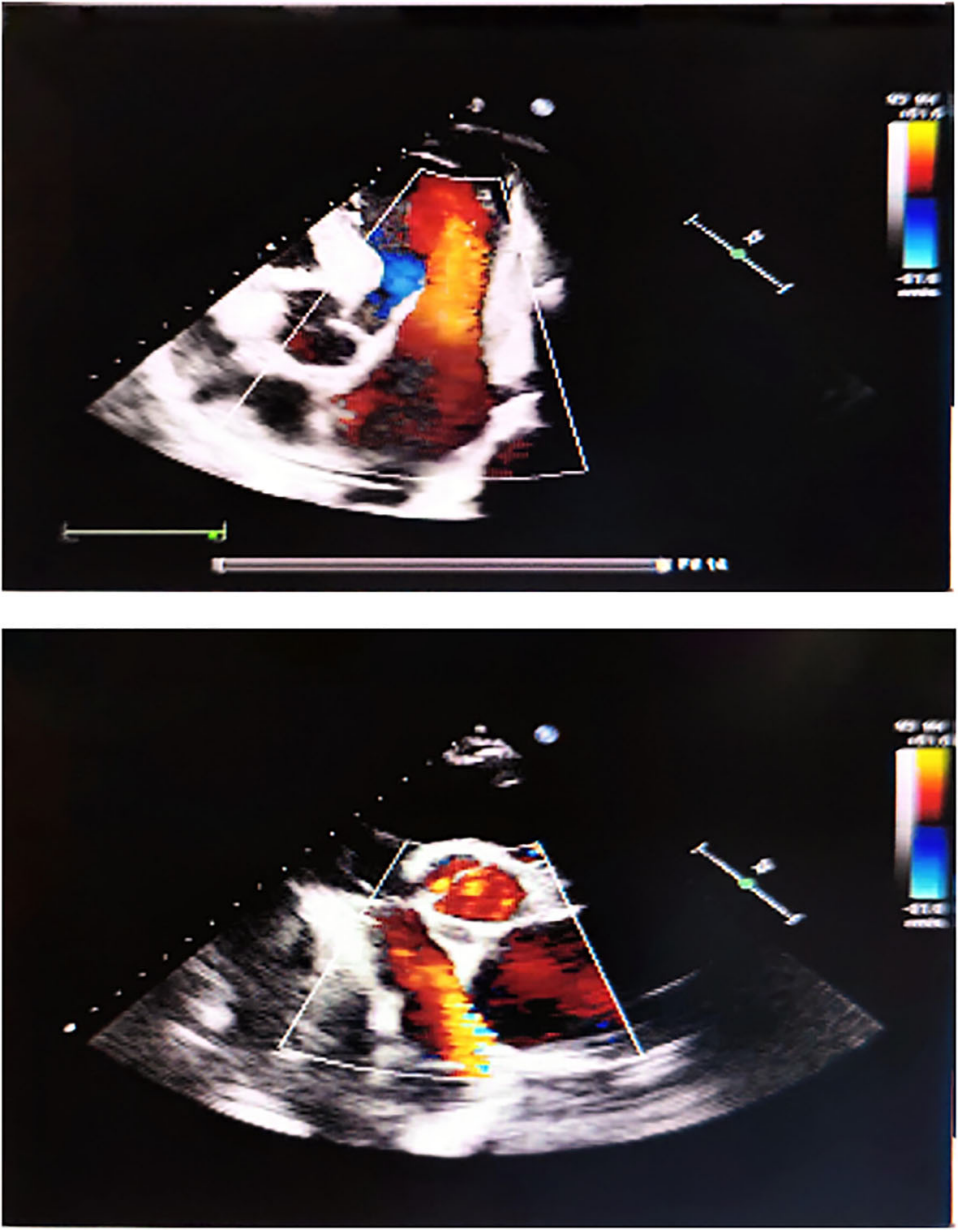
Echocardiography suggests left atrial enlargement.
